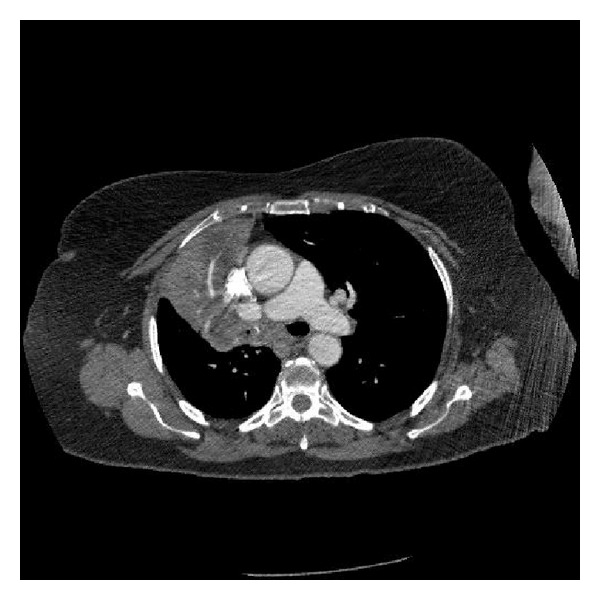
CT scan showing a right hilar mass which encases and narrows the right upper lobe bronchus and the rightmain stem bronchus.
